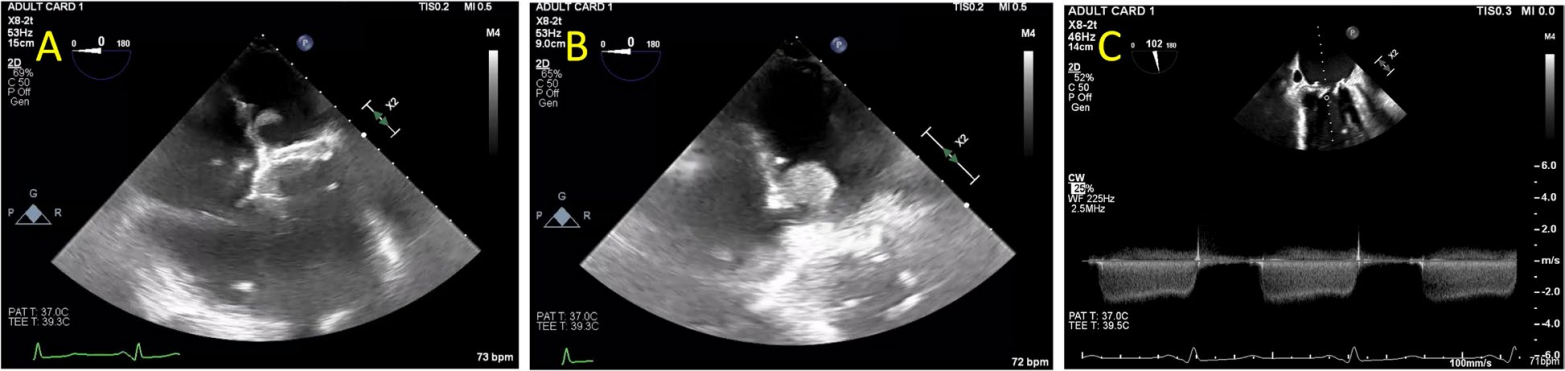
Left atrial thrombus visualized on echocardiogram in mid-esophageal 4 chamber view of bioprosthetic mitral valve. Transesophageal echocardiogram (TEE) demonstrating left atrial thrombus coming into view in end-diastole the (A) mid-esophageal 4 chamber view at 0 degrees, (B) magnified mid-esophageal 4 chamber view at 0 degrees, and (C) continuous wave Doppler across the mitral valve with dense mitral stenosis envelope. Velocity-time integral mean gradient was 19 mmHg at 71 beats per minute
